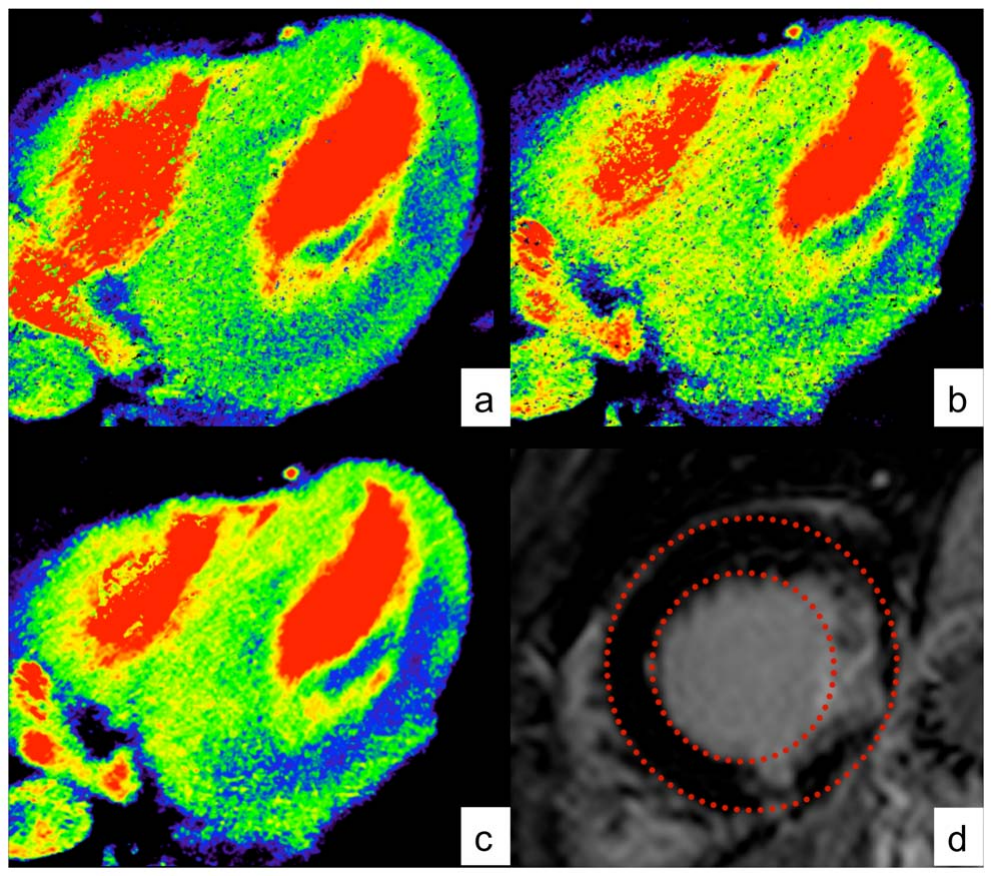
Coloured perfusion maps for original data (a), motion corrected data (b) and combined motion-corrected and spatio-temporal filtered data (c) of Patient 1 in axial orientation. As a reference, short axis view late enhancement MR images are shown (d) presenting partly transmural infarction of the inferolateral wall. LV endocardium and epicardium are outlined in red. The perfusion defect of the inferior and lateral LV wall is visible in all CT perfusion images, with improved delineation of the hypoperfused and remote myocardium using motion correction (b) and best image quality using combined reconstruction algorithm with motion correction and spatio-temporal filtering (c). Thus the transmural extent of the infarction as diagnosed in MRI (d) can be distinguished only in combined motion-corrected and spatio-temporal filtered images (c).
